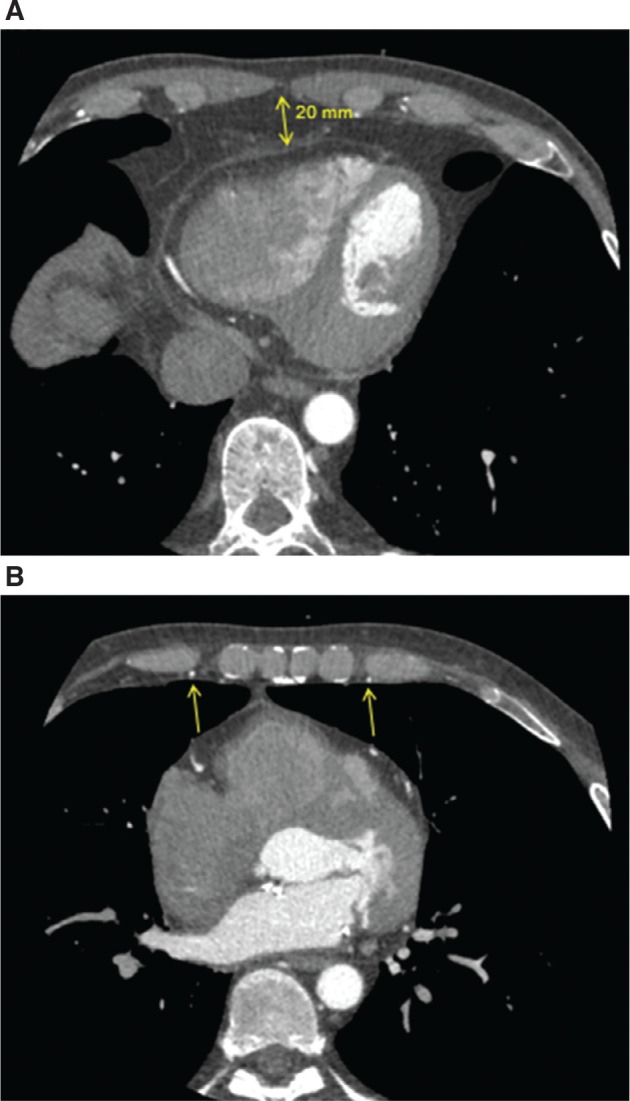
A: A chest computed tomography scan showing the distance between the posterior border of the sternum and the anterior cardiac structures. B: Note the internal mammary arteries are not in proximity to the lateral border of the sternum.
